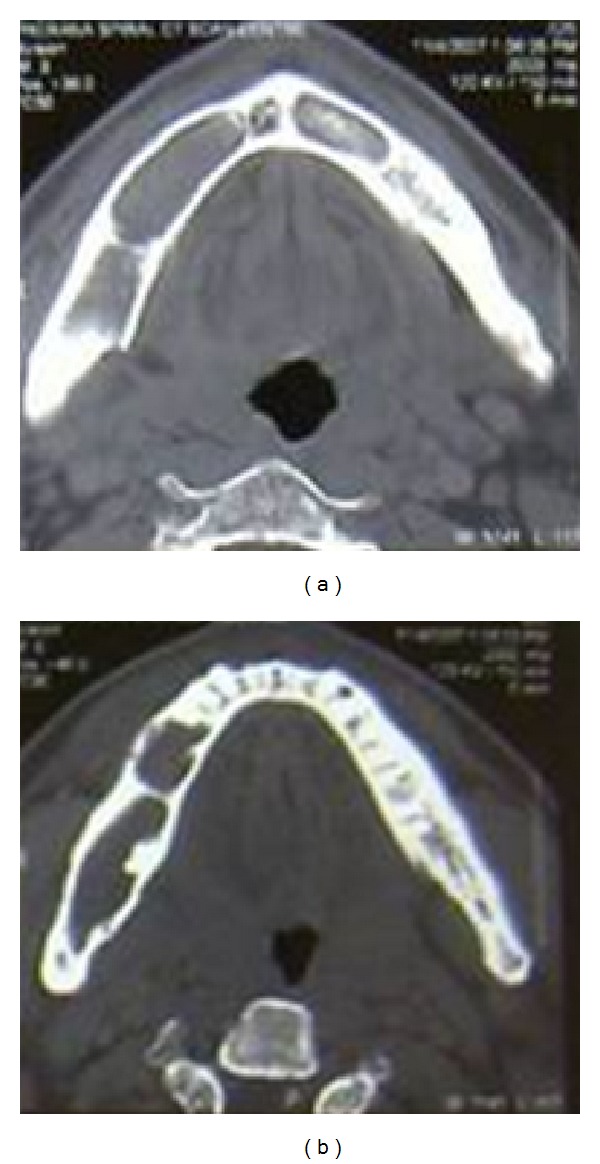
CT scan showing cystic lesions of body and condyle of the mandible.
